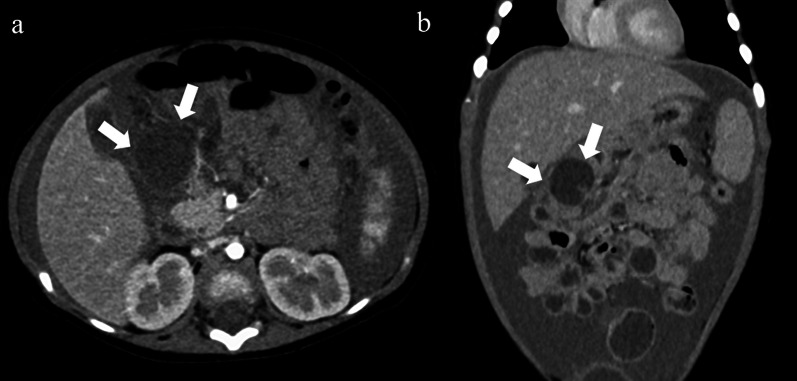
Enhanced CT findings. Enhanced computed tomography showed a cystic lesion (white arrow) anterior to the second portion of duodenum with ascites, as seen in the axial (a) and coronal (b) views
